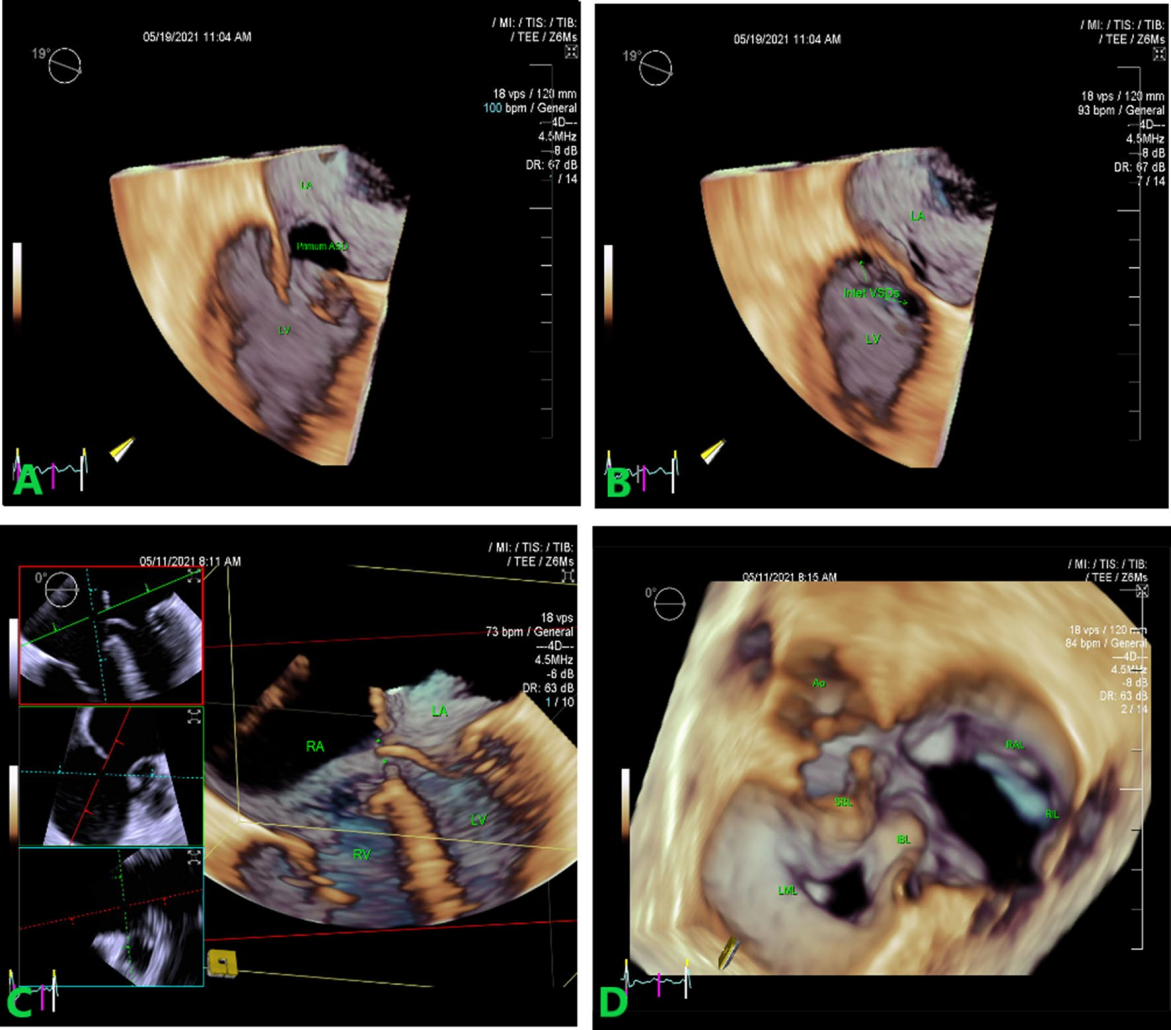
A AVSD—AV septum deficiency. 3D TEE. 3D high volume, high resolution, and single-beat sample, cropped form the front using the green plane adjustment reveals the AV septum deficiency (asterisk). B 3D echocardiography of the primum atrial septal defect—left sided en face view. C Inlet VSDs. LV en face view of inlet interventricular septal defects. Smaller defects (asterisks) in membranous part of IVS. D RT3D TEE left atrial perspective view of the common atrioventricular valve. The common AV valve is with one annulus and two orifices. Both the superior and inferior bridging leaflets are seen. Triangle appearance of the left sided orifice and the elliptic shape of right sided AV orifice. The common valve consist of five leaflets—superior bridging leaflet (SBL), inferior bridging leaflet (IBL), left mural leaflet (LML), right mural or inferior leaflet (RML) and right anterior or superior leaflet (RAL). *AVSD* atrioventricular septal defect, *TEE* transesophageal echocardiography, *VSD* ventricular septal defect, *AV* atrioventricular
